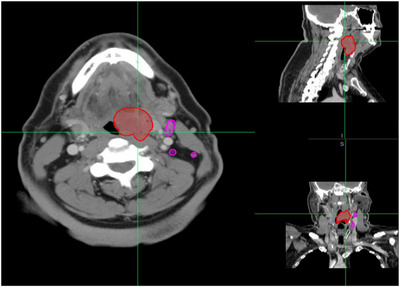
An example of the primary tumor and the lymph nodes falling in interest on a CT scan.
